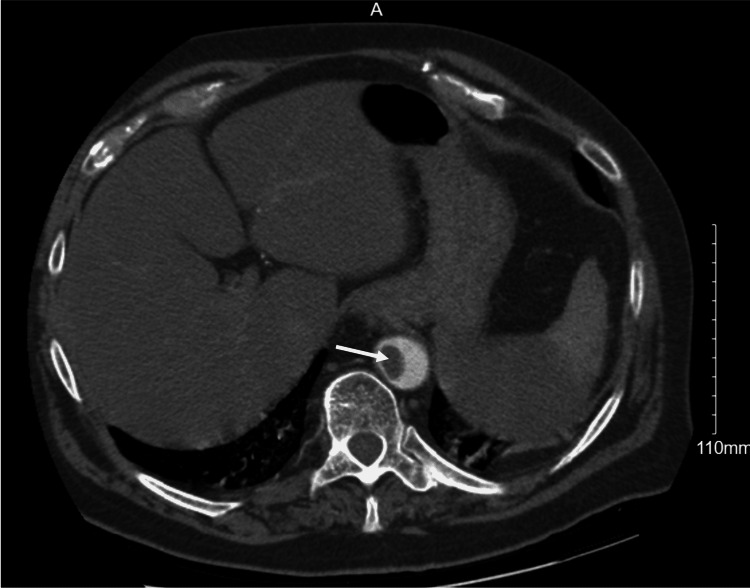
Computed tomography angiogram of the chest showing intraluminal thrombus in the distal thoracic aorta.
